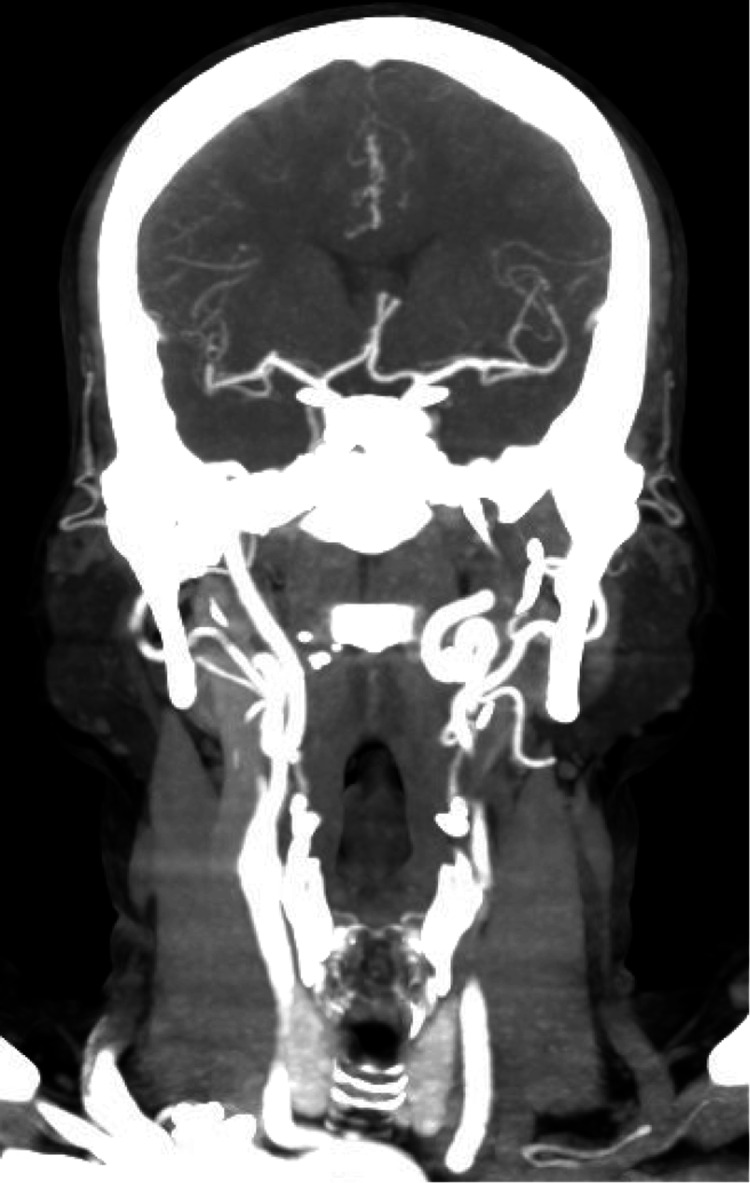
CT angiography showing marked elongation and tortuosity of the bilateral internal carotid arteries
